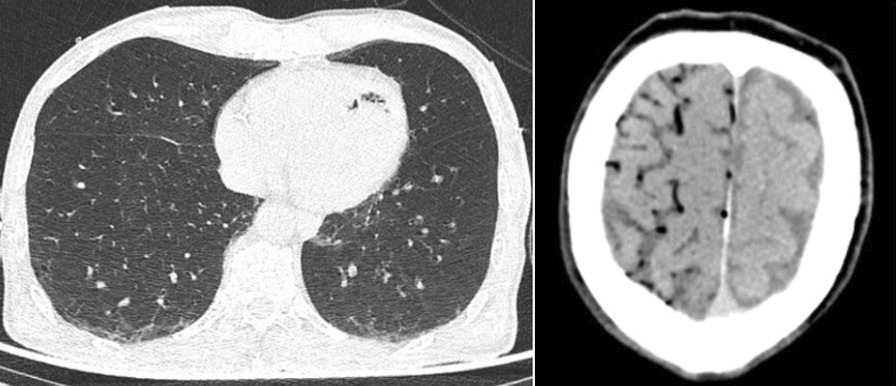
On the left side a thoracic CT-scan showing air emboli in the left ventricle; on the right side a brain CT-scan showing SAE in the bi-hemispheric subarachnoid space of the high brain convexity.
